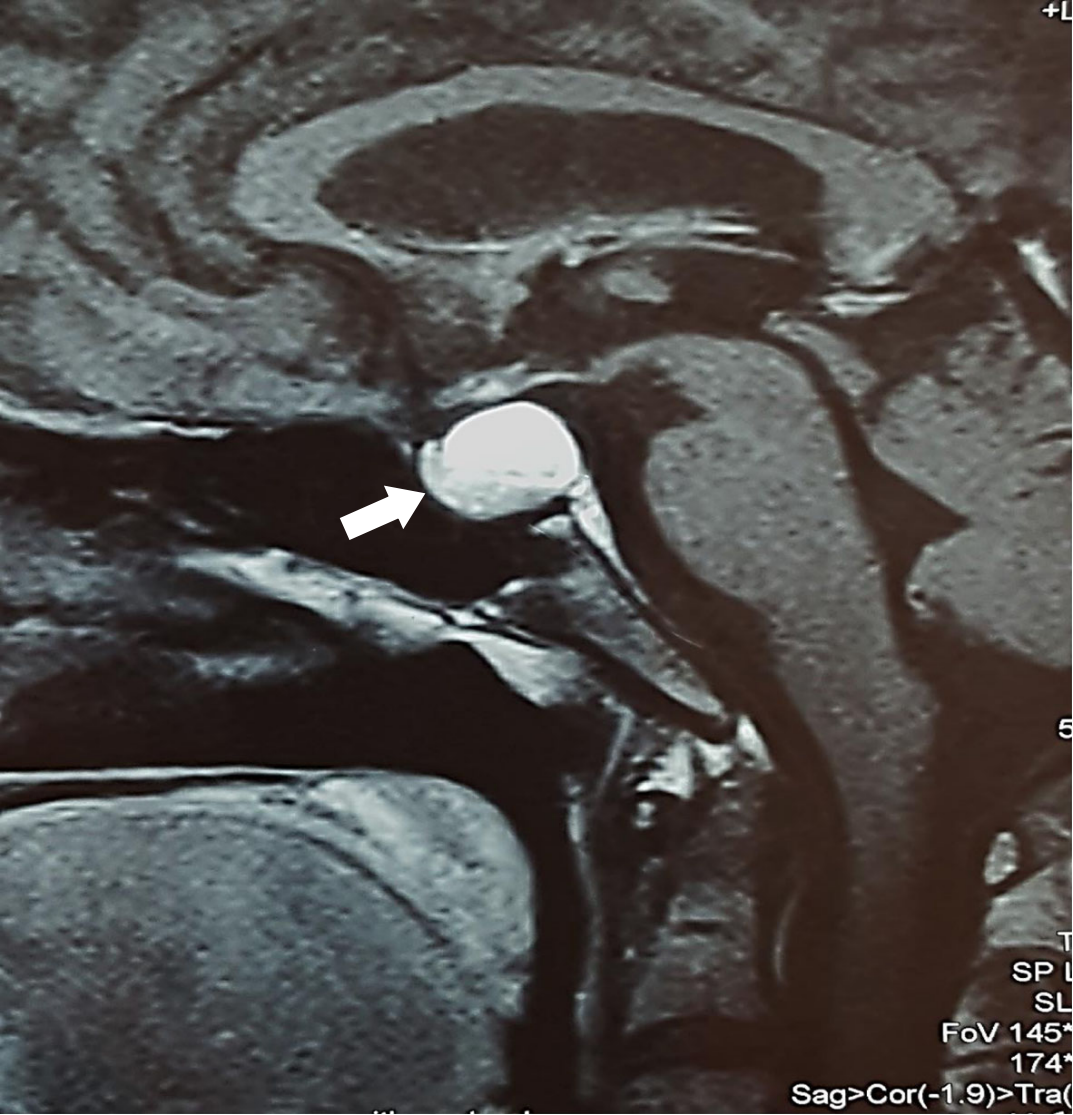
T1-weighted contrast-enhanced magnetic resonance imaging of the brain demonstrates a well-defined craniopharyngioma (*arrow*) within the pituitary fossa with suprasellar extension. The pituitary gland is compressed and the pituitary stalk is displaced by the tumor
